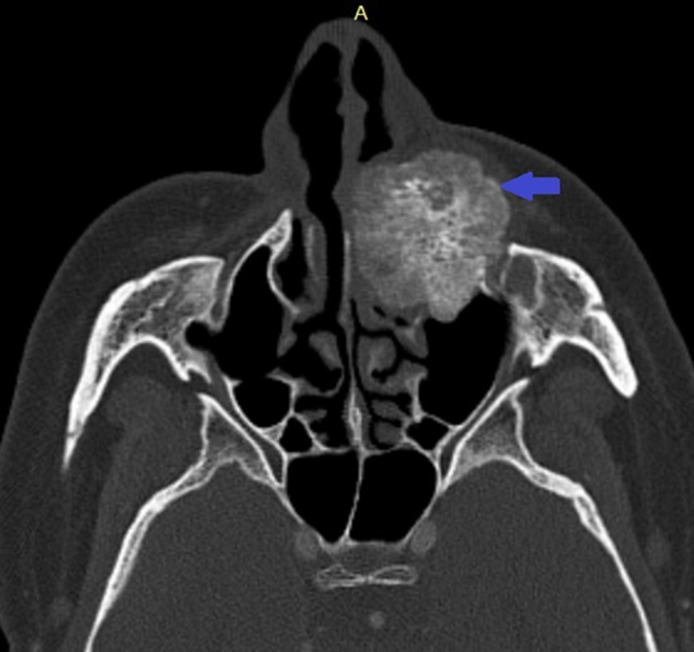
axial CT scans, viewed at wide window settings showing an intraosseous hemangioma arising from the left maxilla (blue arrow)
